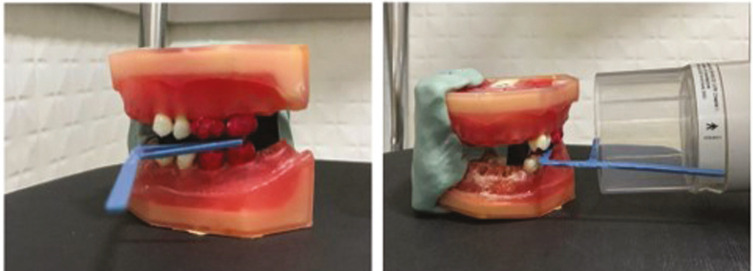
Positioning of the equipment for performing the interproximal radiographic technique.
